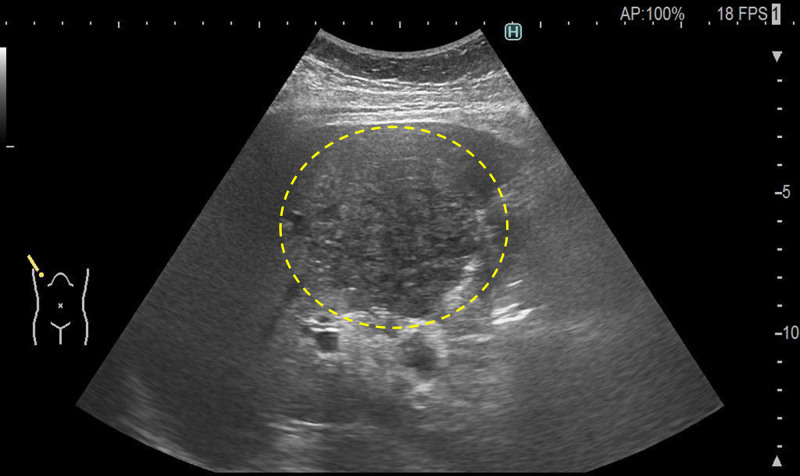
Ultrasonography showed a 6-cm hypoechoic lesion with indistinct margins in the right liver lobe.
